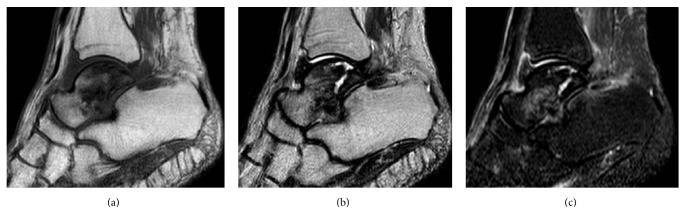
Preoperative MRI showing heterogeneous low signal intensity in the talar body on a T1-weighted image (a), a mixture of low and high signal intensity in the talar body on a T2-weighted image (b), and partial high signal intensity in the talar body on a STIR image (c).
